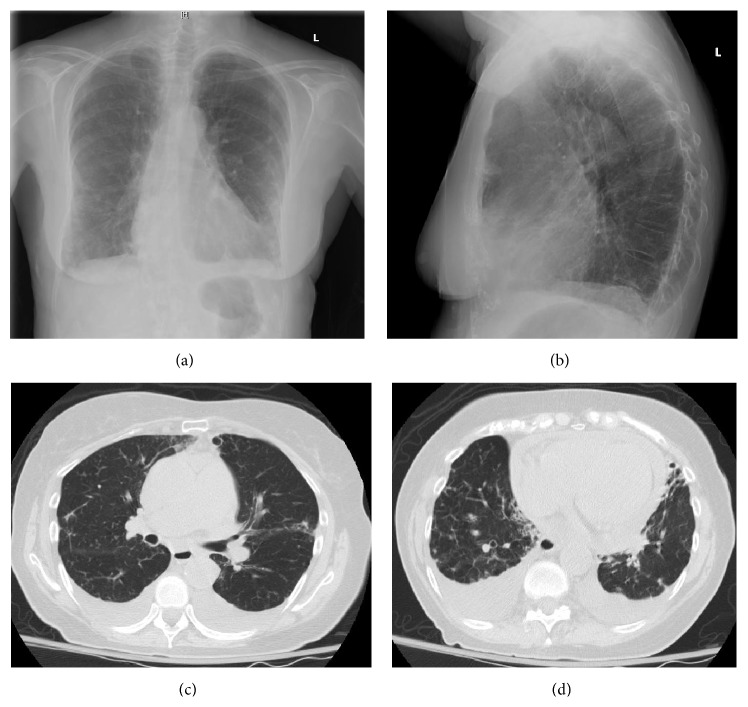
Chest X-ray and chest CT findings in Case 3. Chest X-ray showing stable bilateral nodular opacities and lower lobe predominant interstitial reticular thickening with new small bilateral pleural effusions (a, b). CT of the chest reveals bronchiectasis in the right middle and lingular lobes (c) and tree-in-bud nodular infiltrates in the bilateral lower lobes with new bilateral moderate pleural effusions.
